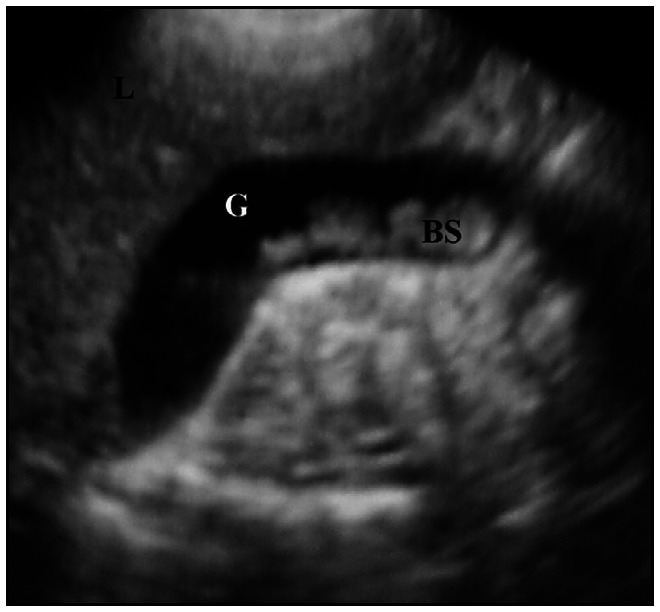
Ultrasound appearance of biliary sludge. G = Gallbladder, BS = Biliary sludge, L = Liver.
